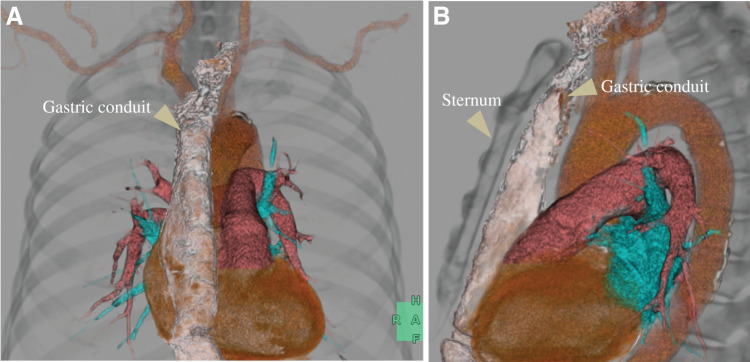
Bone-removed 3D CT demonstrating the gastric conduit behind the sternum (gray arrows). (A) Anterior view. (B) Left lateral view.
